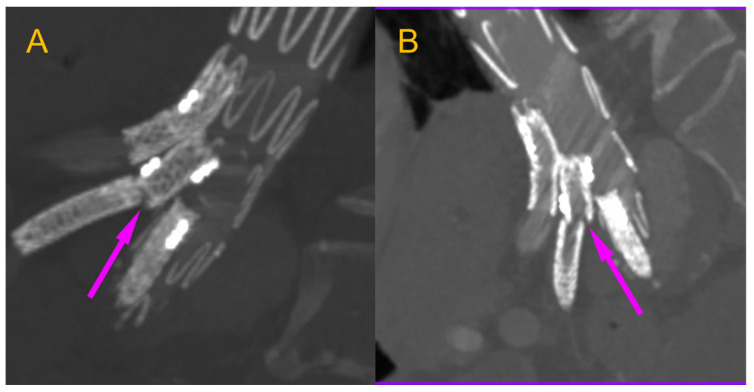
Ruptured superior mesenteric artery branch of stent graft—curved planes. The fractured site is marked with an arrow. Panel (A)—first projection, panel (B)—second projection. Pre-interventional Angio-CT Curved MPR, arterial phase.
